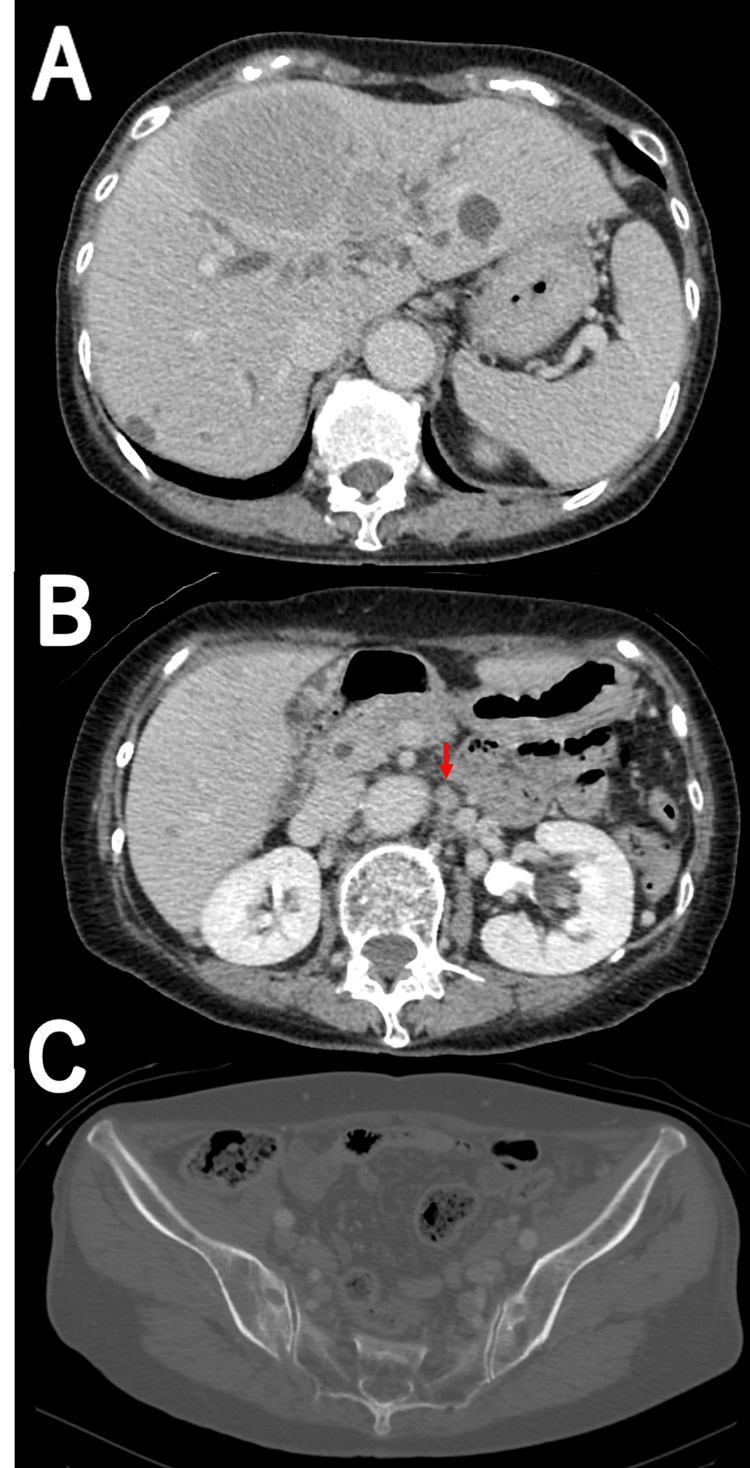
CT scan from a previous hospital. (A) CT scan shows multiple masses in the liver and dilated intrahepatic bile ducts; (B) para-aortic lymph nodes are enlarged (red arrow); (C) the cortex of the right iliac bone appears continuous.
